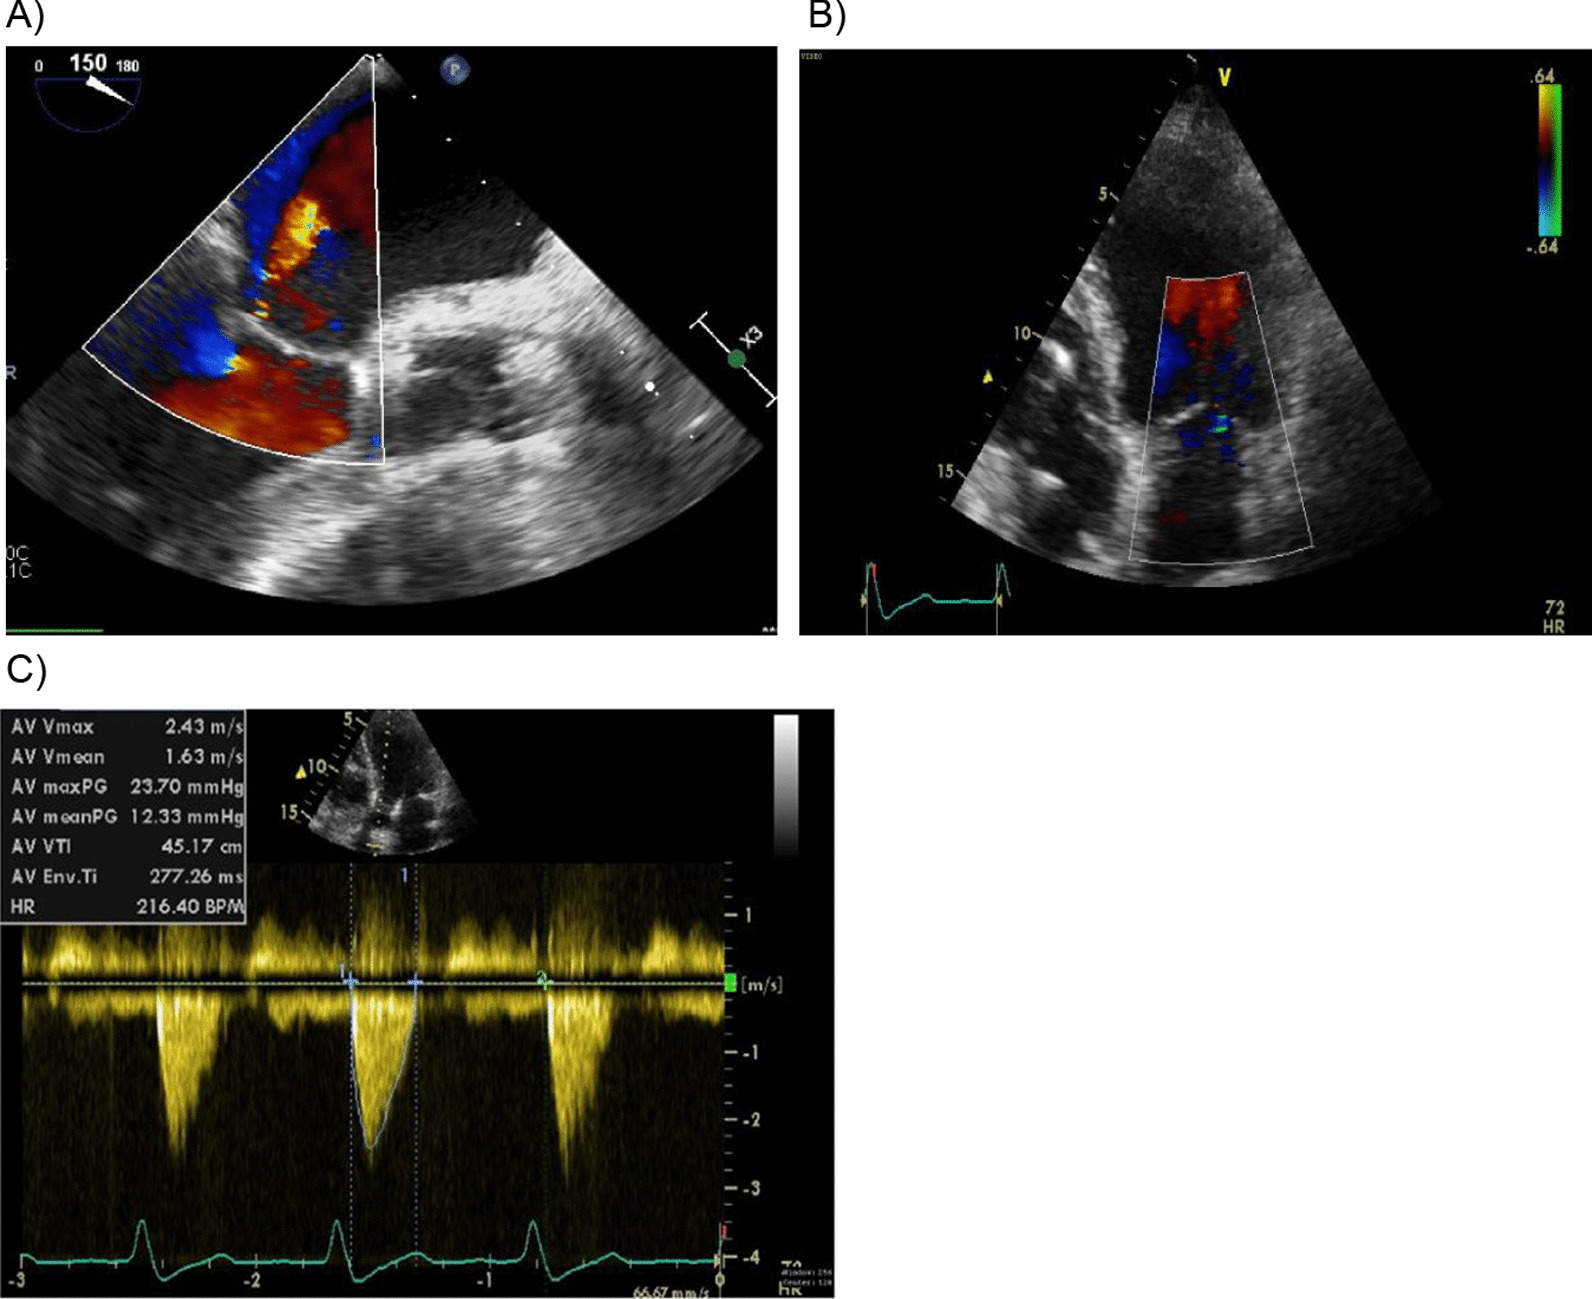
Control Echocardiograms after using suture-less bio-prosthesis (end-results). A Perioperative control showing small regurgitation of the mitral valve. B Restoration of the mitral valve geometry and function after 1 year. C Hemodynamic parameters of suture-less prosthesis after 2 years
